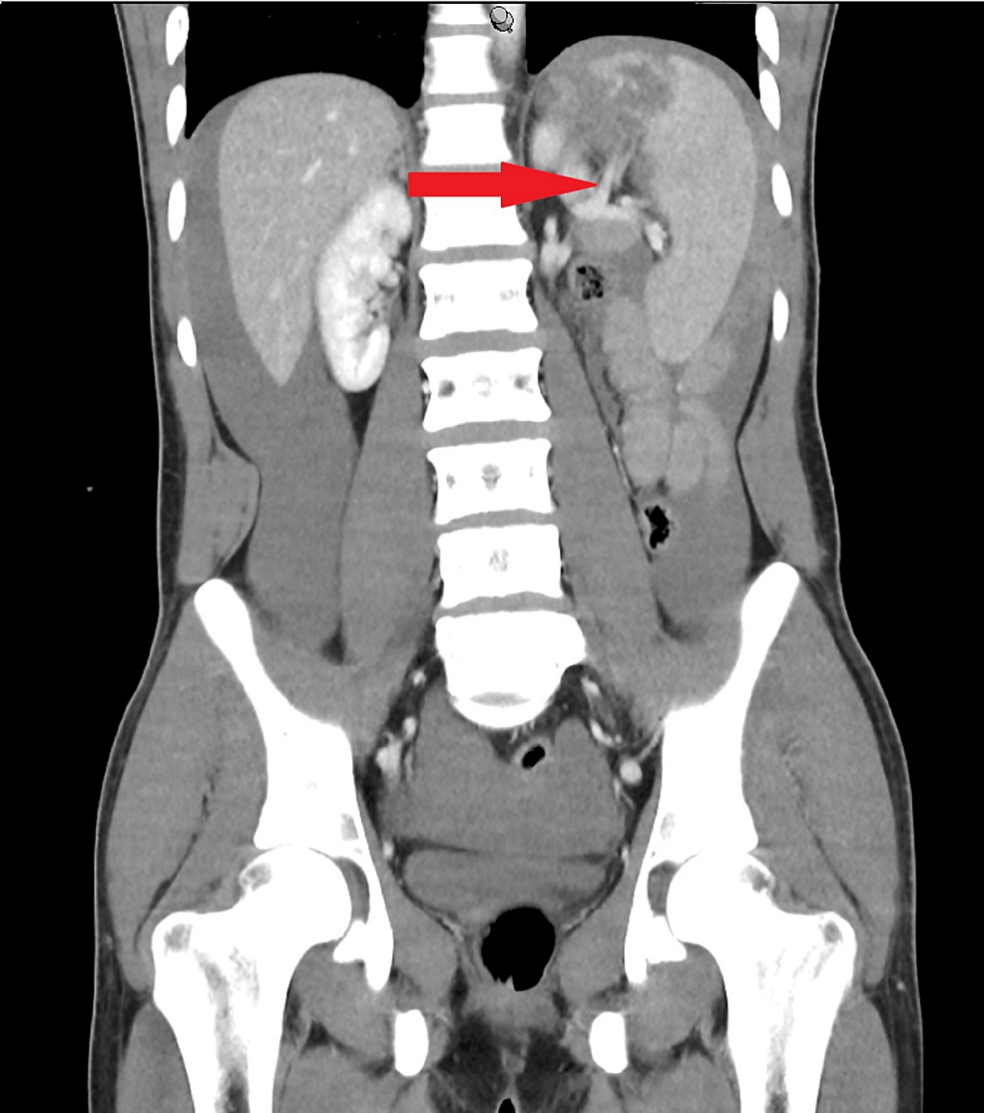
Abdominal CT scan showing splenic injury and involvement of the upper-lobar branch of the splenic artery Red arrow: Upper-lobar branch of the splenic artery extending into upper pole laceration and area of devascularisation
